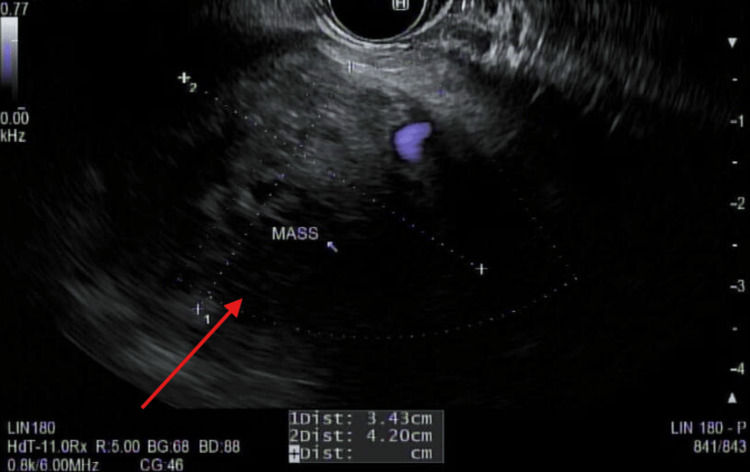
EUS revealing an oval, hypoechoic mass in the tail of the pancreas measuring 4.2×3.43 cm in maximal cross-sectional diameter Arrow highlights the well-defined margins of the hypoechoic pancreatic mass. EUS: endoscopic ultrasound
